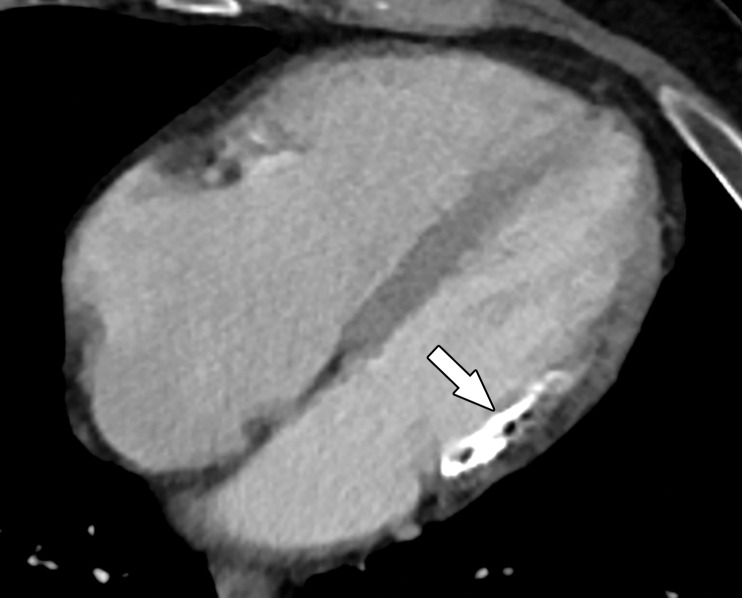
Four-chamber (a) and short-axis (b) CT images in a 65-year-old man show an area of hypoenhancement and calcification within a thinned nonenhancing basal inferolateral wall (arrow), compatible with a chronic MI. This MI was incidentally detected on a routine nongated contrast-enhanced chest CT image obtained for chronic dyspnea.
